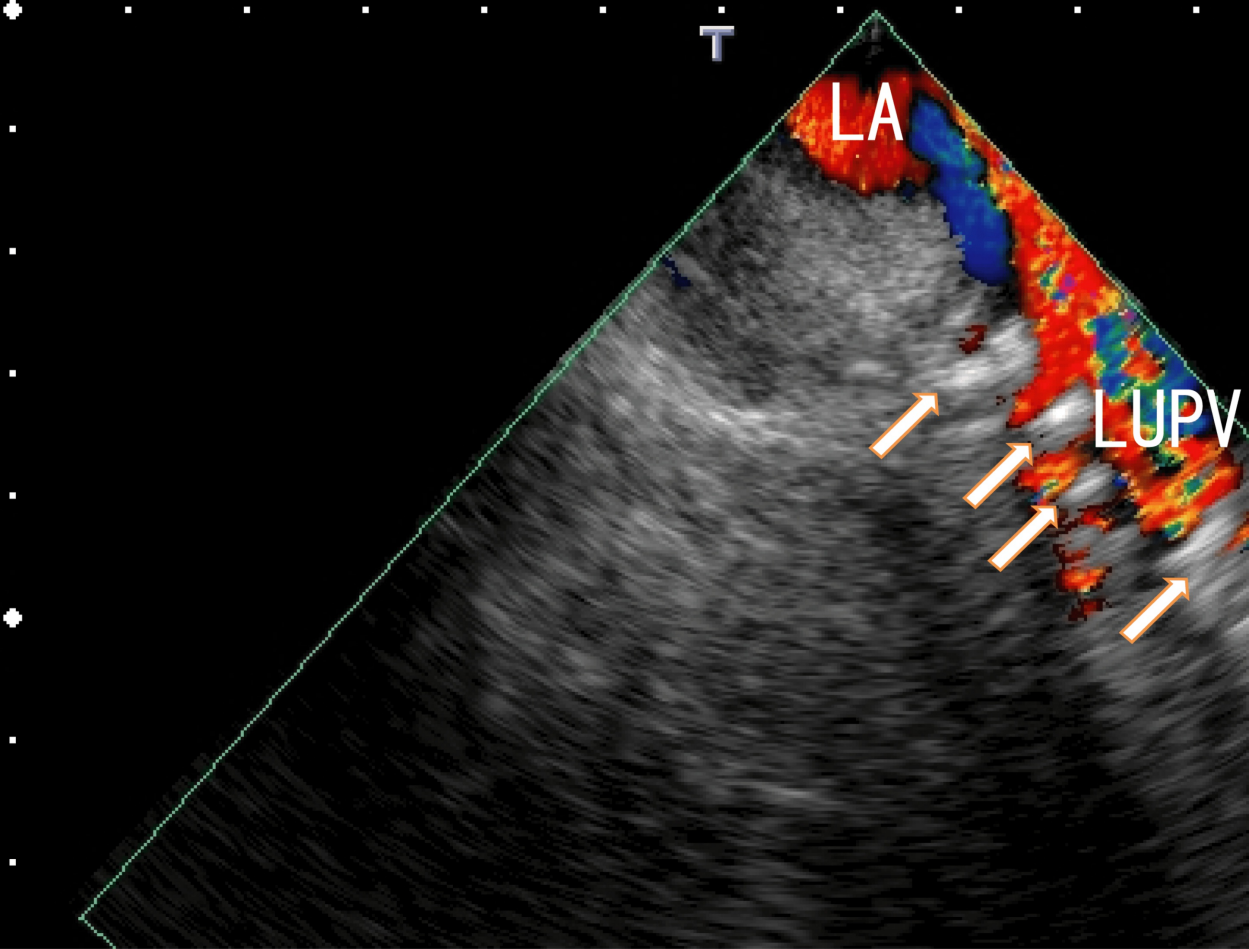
Transesophageal echocardiography (TEE) images showing thrombi TEE images showing thrombi in the left upper pulmonary vein (LUPV) (arrows). The thrombi are attached to the wall of the LUPV and present as several hyperechoic regions. The average size of the hyperechoic regions measured approximately 2 mm × 5 mm and were arranged perpendicularly to the direction of the LUPV. LA: left atrium, LUPV: left upper pulmonary vein
